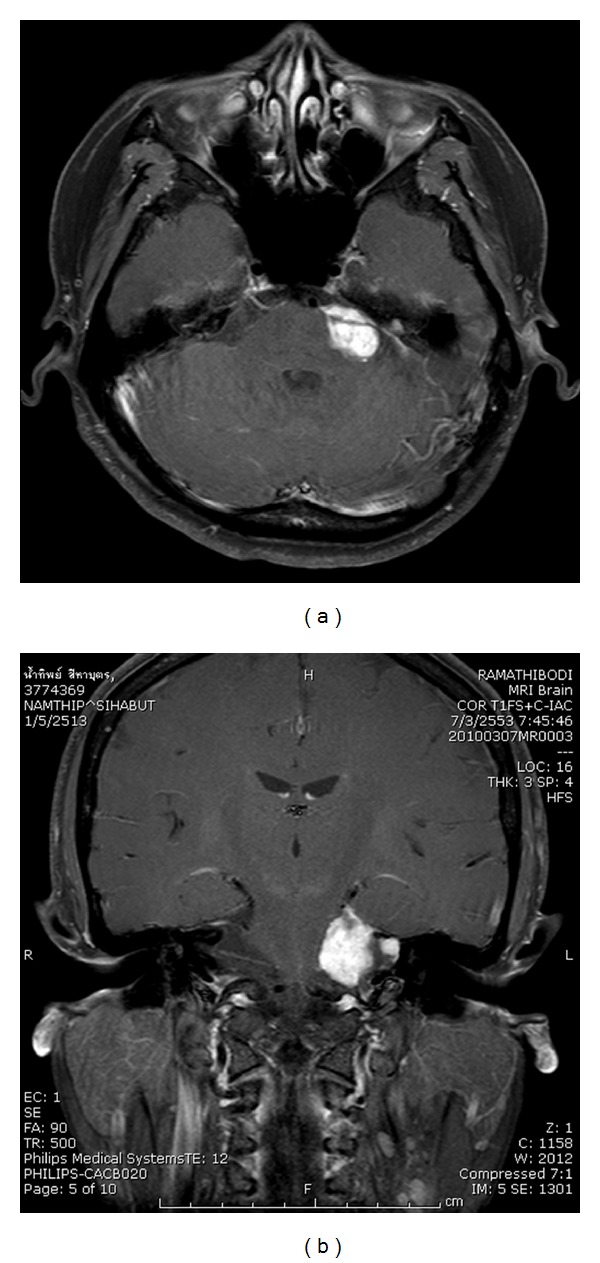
Axial and coronal T1W MRI with contrast showing a left vestibular schwannoma at the time before SRT (2005).
